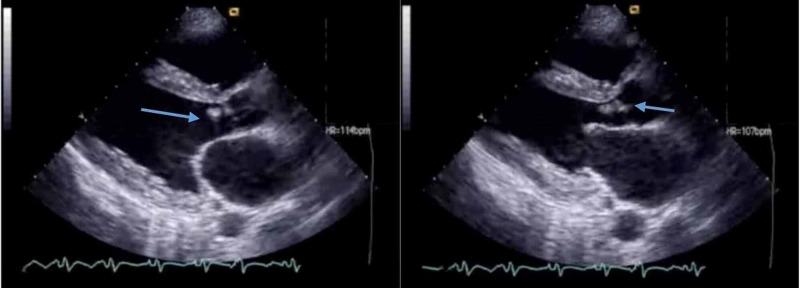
Echocardiography showing aortic valve vegetations consistent with possible infective endocarditis (blue arrows).
